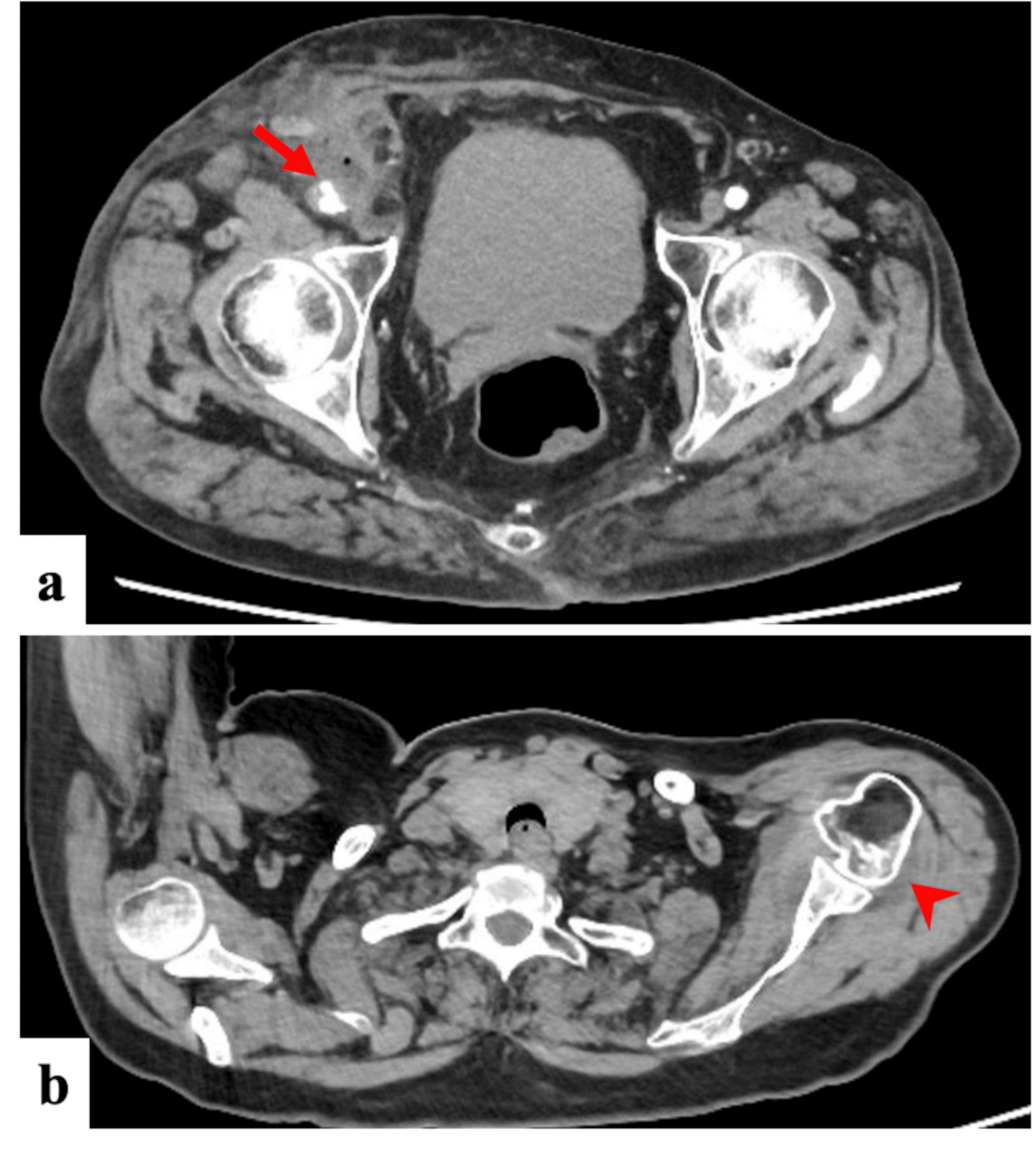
Initial computed tomography scans: infected pseudoaneurysm and contralateral septic arthritis (a) Contrast-enhanced computed tomography imaging of the right groin showing a 5-mm protrusion (arrow) on the ventral side of the right femoral artery with surrounding inflammatory changes including increased fat tissue density, internal gas formation, and regional lymphadenopathy, consistent with infected pseudoaneurysm formation. (b) Non-contrast computed tomography of the left shoulder demonstrating a low-density area around the left subscapularis muscle (arrowhead) with extension into the glenohumeral joint space, suggesting synovial fluid accumulation and septic arthritis.
